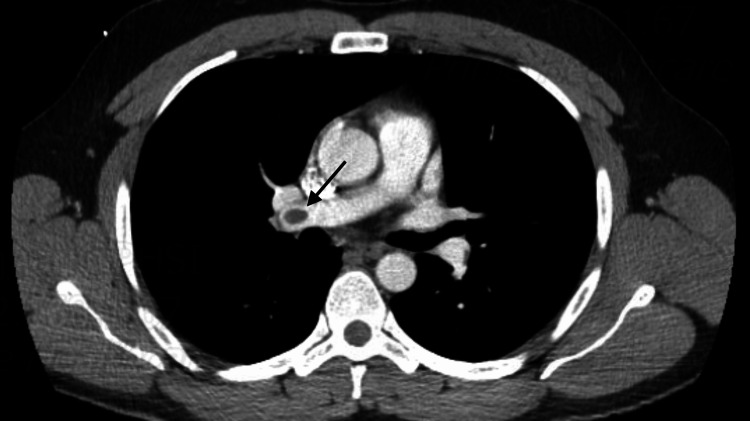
The CT angiogram of the chest with and without contrast showing pulmonary embolism CT: computed tomography
